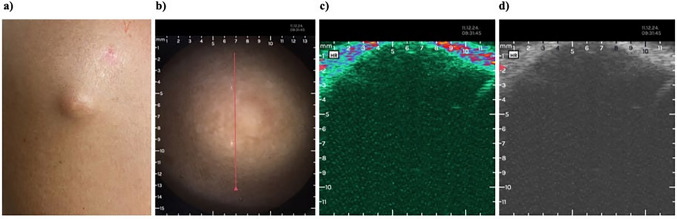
Epidermoid cyst in a 56-year-old female patient, Fitzpatrick skin type II. a Clinical image showing an oval-shaped, regular, raised, skin-coloured lesion located on the right deltoid region. b Dermoscopic image of the lesion. c, d Dermoscopy-guided high-frequency ultrasound (DG-HFUS) images demonstrating a dermal lesion with a measured depth of 2.8 mm. The lesion appears oval, well-defined, and sharply demarcated lesion that is predominantly hypoechoic with small internal hyperechoic areas and bands, consistent with keratin fibres
